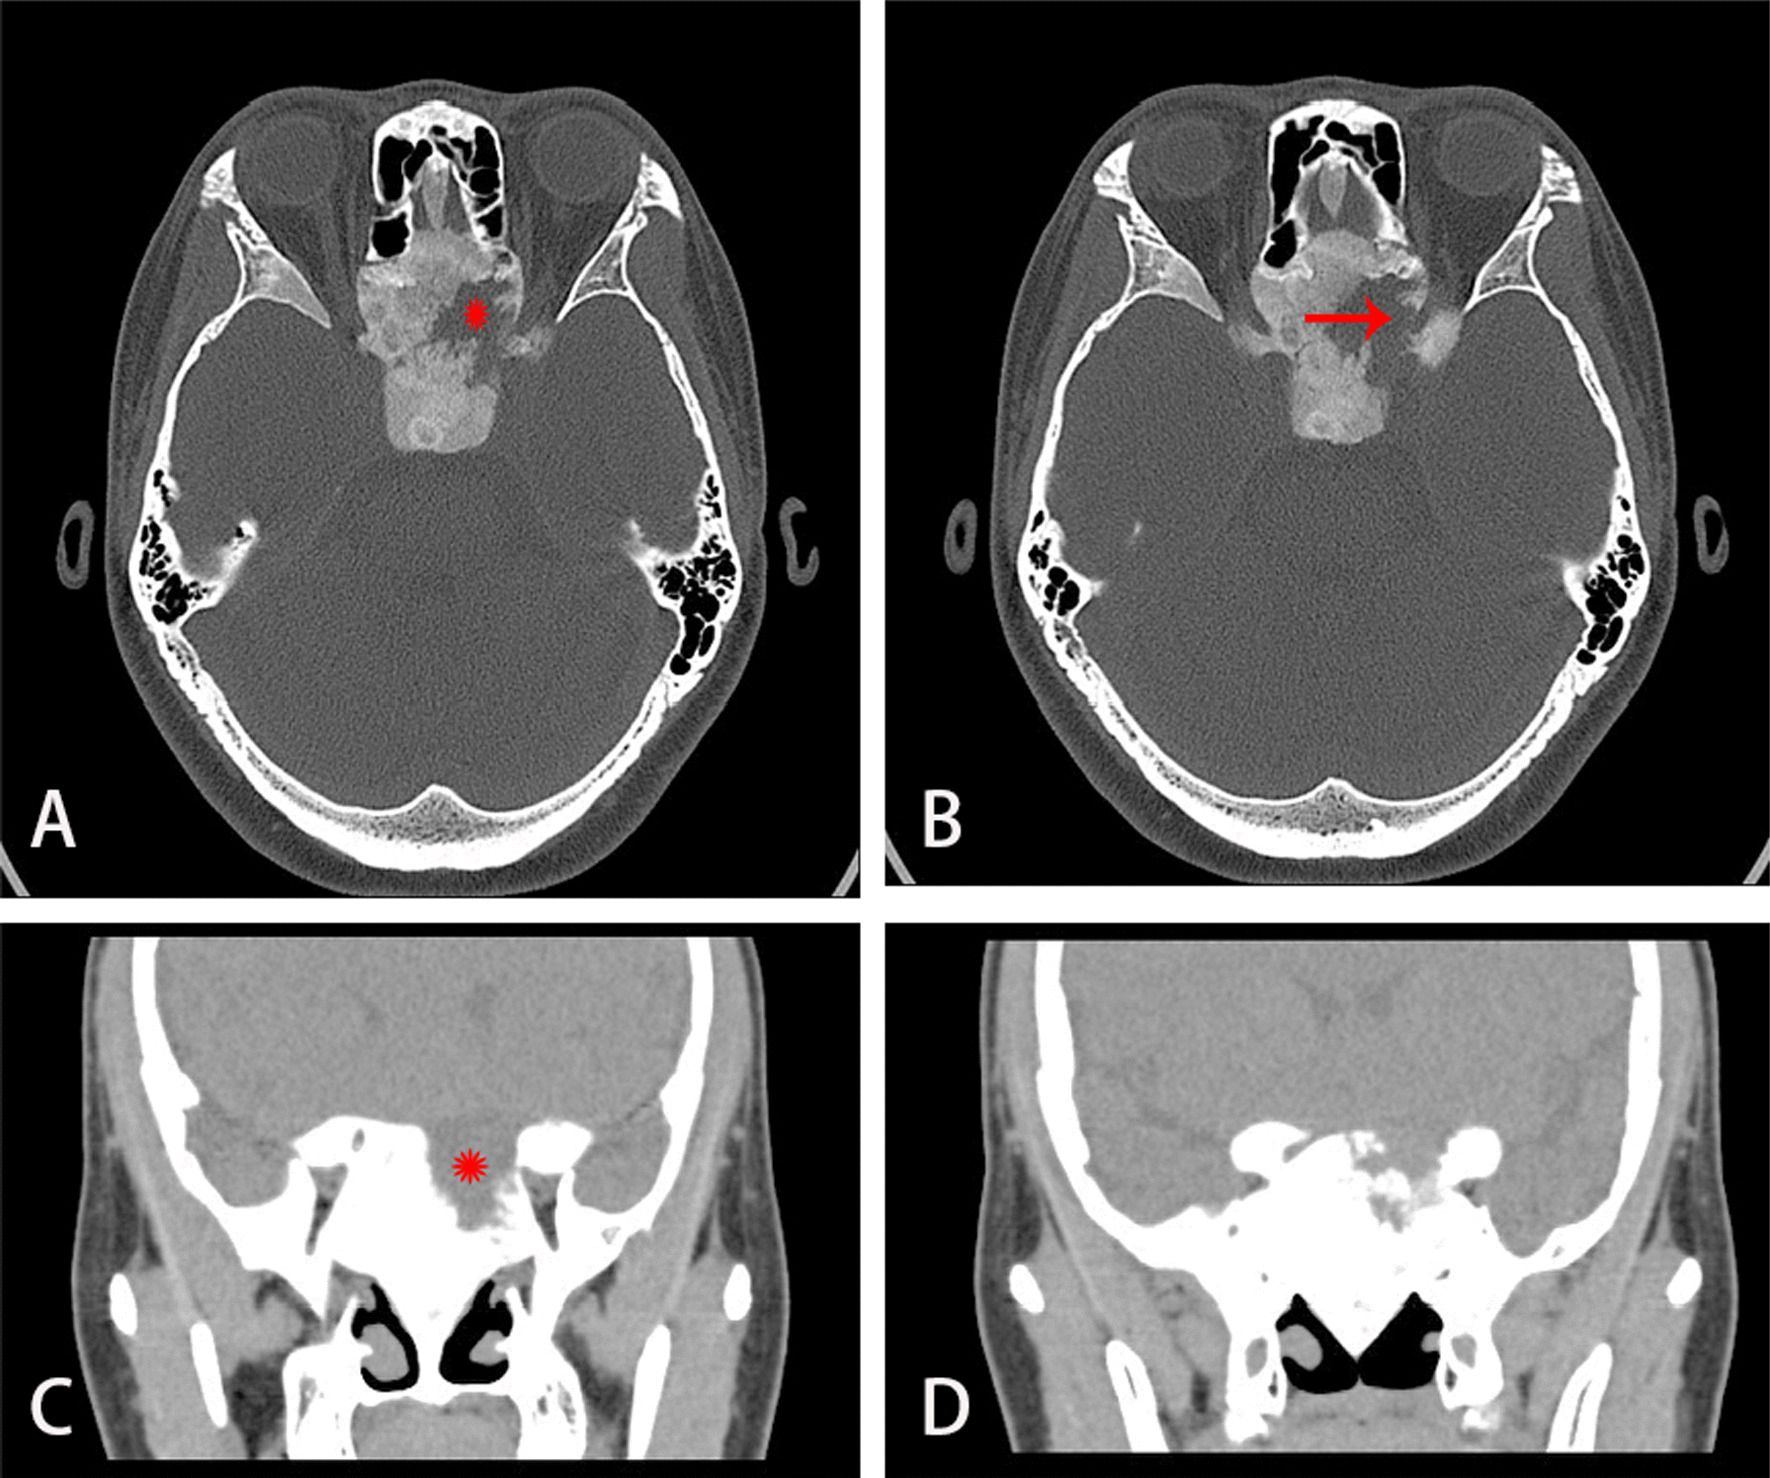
Orbital CT before surgery. A, C Orbital CT indicates fibrous dysplasia of bone with cystic degeneration (red star sign). B red arrow indicates optic nerve. D Shows the overall situation in CT
